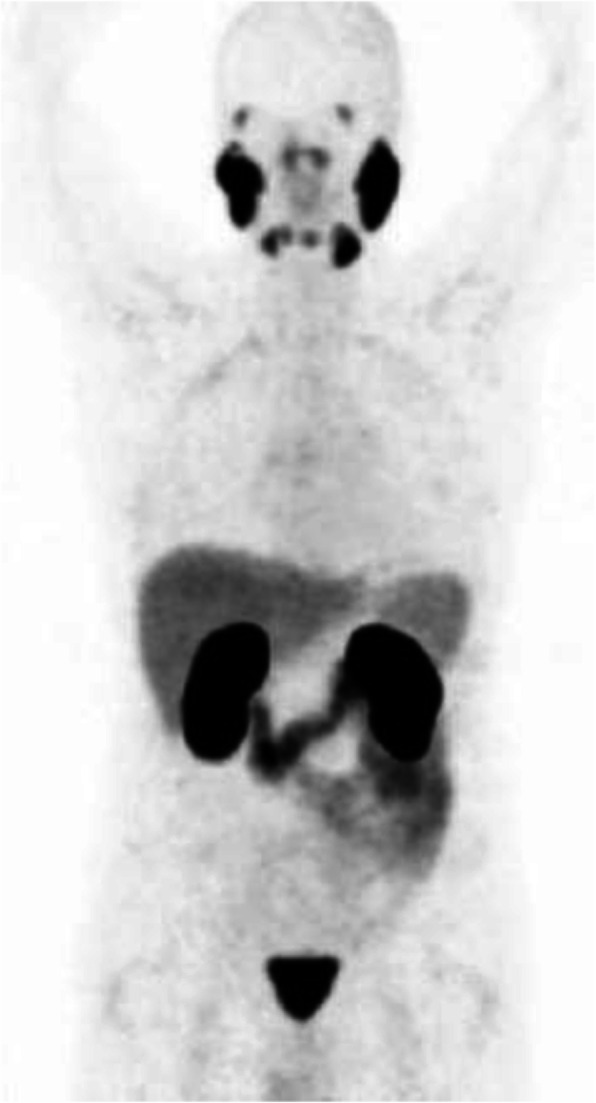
Physiological biodistribution of ^68^Ga-PSMA (PSMA 11). Salivary glands, small bowel (jejunum), kidneys, spleen, and liver are the organs with the highest uptake of ^68^Ga-PSMA-11
